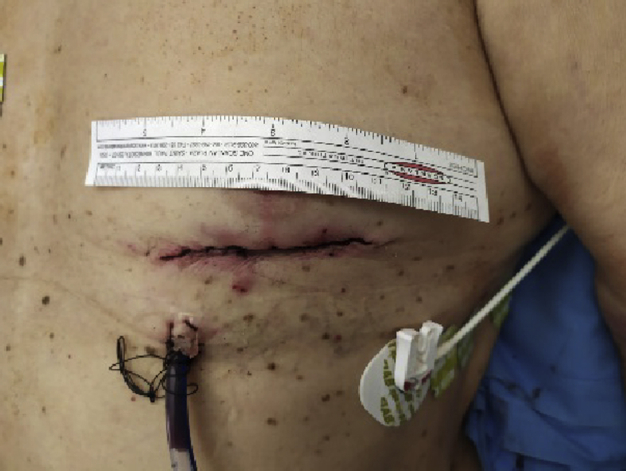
Left ventricular access used for the first transapical procedure on CT scan.
